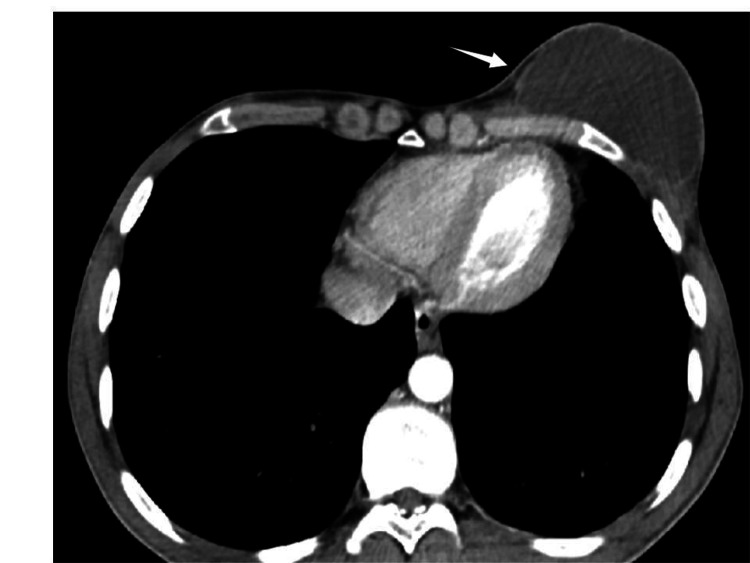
Enhanced axial slice of chest CT in the mediastinal window demonstrating a well-defined hypodense fluid collection with a thin wall and fluid density on the left superior thoracic wall mimicking a breast prosthesis (white arrow).
